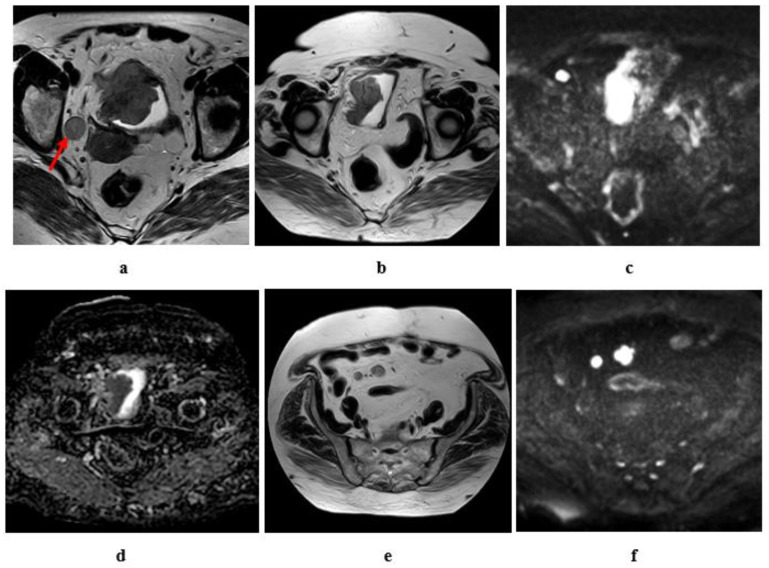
Tumor recurrence eight years after radical cystectomy and ileal-w-neobladder in a female patient, aged 67 years, the primary tumor was SCC, stage T3b N2 M0. (a) High resolution T2w MRI of the pelvis: an intravesical soft tissue mass at the right lateral bladder wall, RT side obturator LN (red arrow); (b–f) 8 years later (post radical cystectomy), (b) high resolution T2 w MRI of the pelvis: tumor recurrence in the ileal-w neo bladder, (c) diffusion w MRI (b value = 1400) revealed restricted signals of the recurrent tumor, (d) ADC of the mass with hypointense SI: 0.9 × 10^−3^ mm^2^/s, (e) high resolution T2 of the lower abdomen: two enlarged mesenteric LNs. (f) Diffusion w MRI ((b) = 1400): restricted diffusion of the mesenteric LNs.
